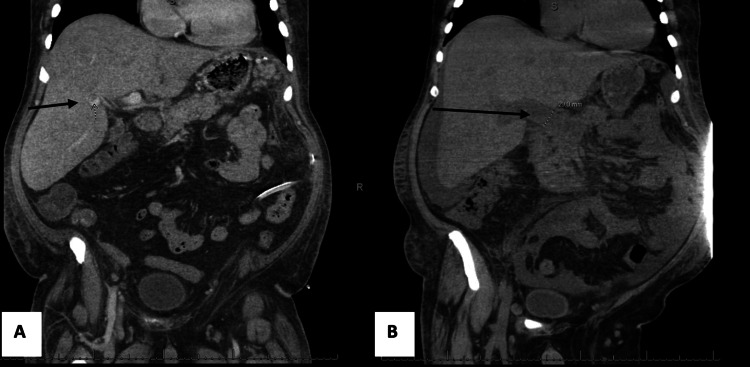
This figure compares two sagittal views of the CT scans of the pulmonary abdomen pelvis taken in January 2023 (A) and April 2023 (B). The images highlight the progression of the portal vein thrombosis over the three-month period. In (B), the entire portal vein and splenic vein are thrombosed with partial thrombosis of the superior mesenteric vein near the portal confluence.
